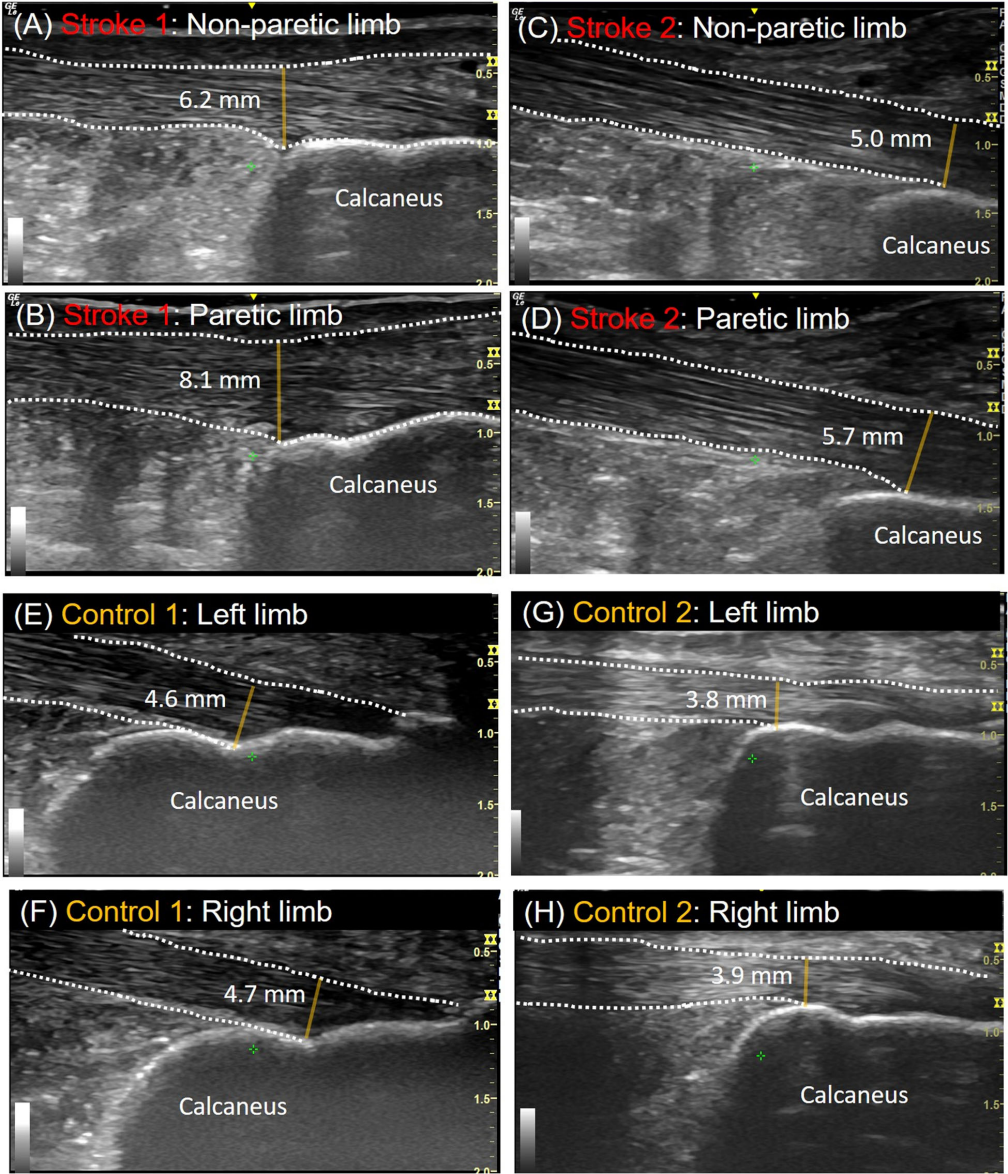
Longitudinal views of the Achilles tendon sonography at the Achilles tendon insertion for a the non-paretic limb of stroke case 1; b the paretic limb of stroke case 1; c the non-paretic limb of stroke case 2; d the paretic limb of stroke case 2; e the left limb of control case 1; f the right limb of control case 1; g the left limb of control case 2; h the right limb of control case 2. The yellow line on each image indicates the thickness of the Achilles tendon. The dash lines highlight the borders of the Achilles tendon
